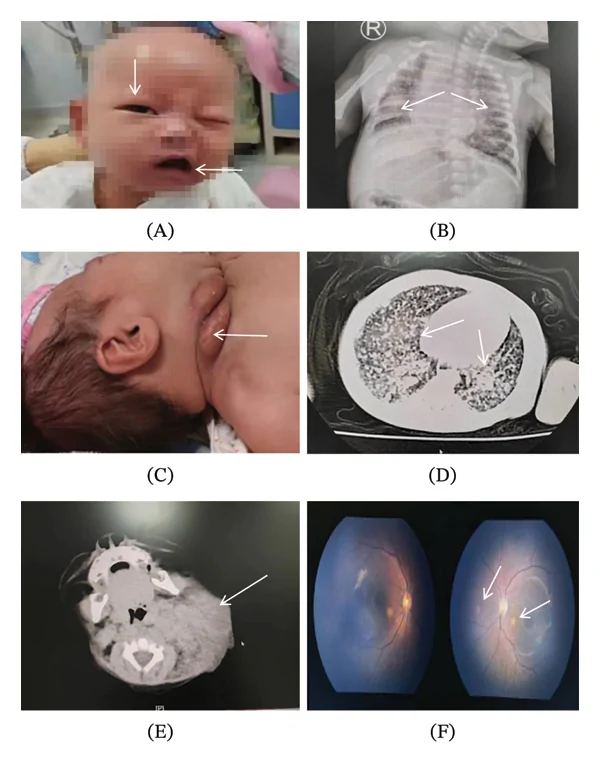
(A) First‐born twin, facial palsy. (B) First‐born twin (PND95), chest X‐ray image exhibiting pneumonia. (C) Second‐born twin, cervical mass. (D) Second‐born twin, pulmonary CT showing miliary changes (PND95). (E) Second‐born twin, CT scan of the neck showed a large cystic mass. (F) Second‐born twin, two yellow lesions in the posterior fundus of the left eye.
